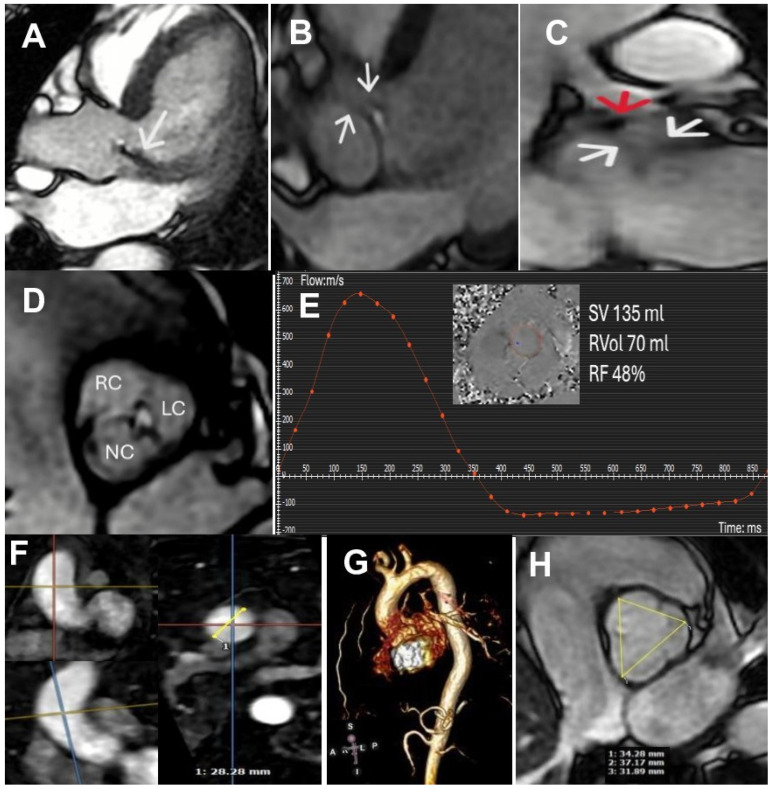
After TEE, aortic regurgitation was classified as severe, and the patient was referred for cardiac surgery, for which cardiac magnetic resonance imaging (cMRI) was performed to assess the thoracic aorta anatomy. Steady-state free precession (SSFP) cine imaging revealed in 3-chamber long-axis view in diastole an eccentric jet of aortic regurgitation visualized by the low signal due to spin-dephasing caused by shear and turbulence (white arrow, panel (A)). The spatial resolution of approximately 1–2 mm is insufficient to clearly visualize the ACTS; however, in the three-chamber long axis cine diastolic image (white arrows, panel (B)) and coronal cine diastolic image (white arrows, panel (C)), hypointense linear structures are observed, causing cusp retraction, and the high origin of the ACTS, at the level of the sinuses of Valsalva, is clearly visible as a focal nodular hypointensity (red arrow, panel (C)). SSFP short-axis cine image (panel (D); Supplementary Video S3) demonstrates asymmetric diastolic retraction of all three cusps, leading to incomplete leaflet coaptation. Finally, the biventricular function was quantified using short-axis SSFP cine images. Specifically, normal biventricular function was observed (LVEF 56%, RVEF 62%), along with moderate left ventricular dilation (EDVi 124 mL/m^2^). Contrast-enhanced MR angiography, utilizing multiplanar reconstructions, allowed for the assessment of the ascending aortic diameter, which was found to be within the normal range (double-oblique technique, panel (F); 3D aortic reconstruction, panel (G)). The aortic sinus-to-sinus diameter measurements fall within the normal range (SSFP cine short axis at aortic level, panel (H)) The quantification of aortic regurgitation was performed using through-plane phase contrast velocity mapping at the level of the ascending aorta. Moreover, cMRI confirmed severe aortic regurgitation, with a regurgitant volume (RVol) of 70 mL and a regurgitant fraction (RF) of 48% (panel (E)). Tissue characterization sequences, including late gadolinium enhancement and mapping, were negative. The patient, therefore, given the anatomical findings and young age, is awaiting surgical aortic valve replacement. cMRI proves to be a fundamental modality in the evaluation of valvular disease. It provides a comprehensive assessment by defining the regurgitation mechanism and quantifying RF and RVol, remaining the gold standard for biventricular function and volume analysis. Additionally, it enables detailed aortic anatomy assessment and myocardial tissue characterization, which has significant prognostic implications [4,5]. To date, this represents the first clinical case describing ACTS using cMRI, demonstrating the utility of this imaging technique in this setting. However, in our opinion, this case underscores the importance of a multimodality imaging approach. In fact, TTE remains the fundamental first-line modality, essential for initial disease characterization, identification of potential mechanisms, and severity assessment of regurgitation, guiding the need for further second-level imaging.
